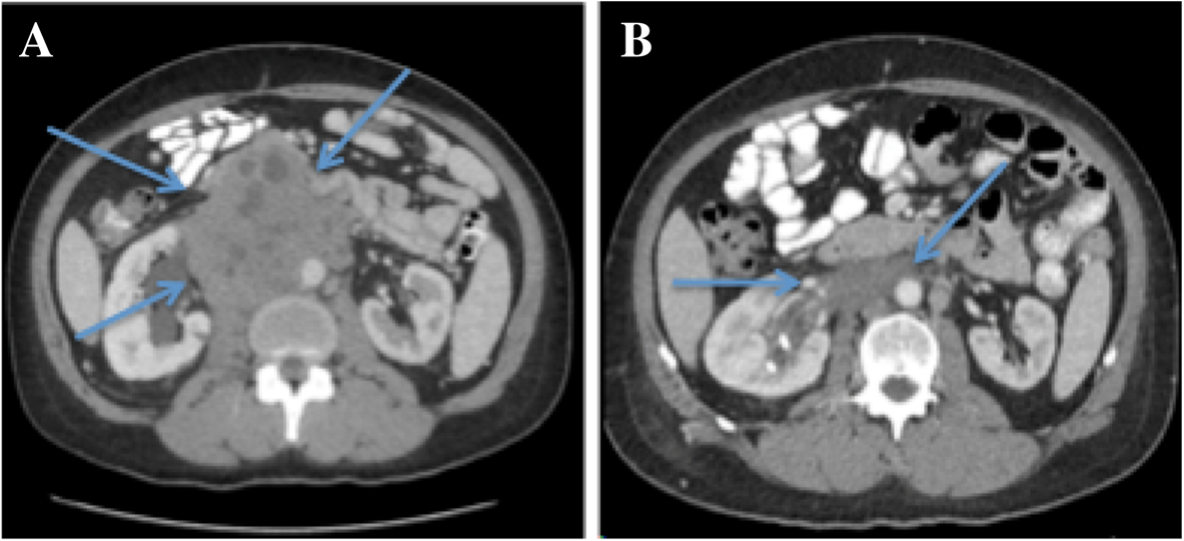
a Pre-treatment computed tomography scan of the abdomen revealing a large, 11 cm retroperitoneal mass causing right-sided hydronephrosis. b Post-treatment (four cycles of bleomycin, etoposide, and cisplatin) computed tomography scan of the abdomen showing significant interval decrease in the retroperitoneal mass. Arrows are pointing to the retroperitoneal mass. Pre and post treatment
